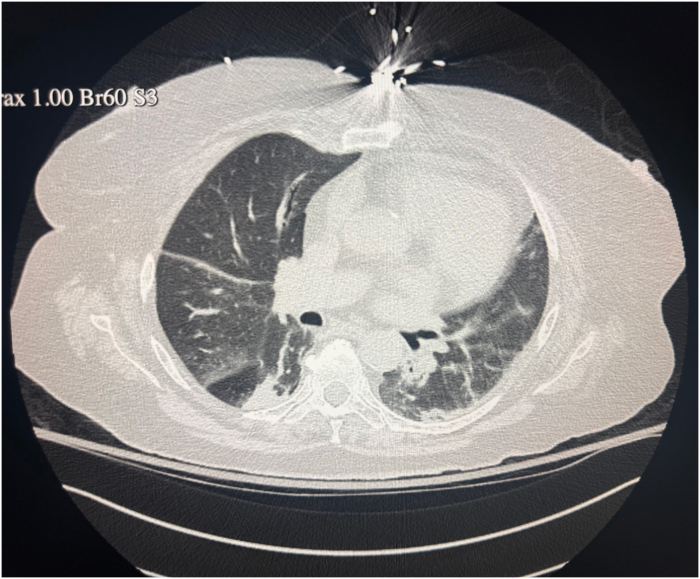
Chest Computed Tomography Demonstrating Bilateral Ground-Glass Opacities Concerning for Immune Checkpoint Inhibitor–Associated Pneumonitis
